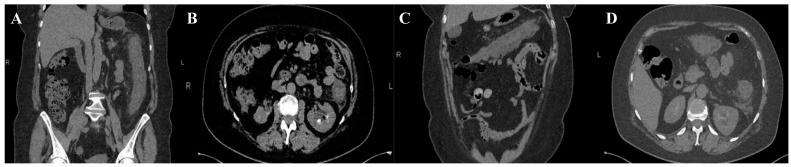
Second abdominal CT. Extensive and continuous lesions, up to hepatic flexure and down to sigmoid with pericolic fat stranding. Coronal (A,C) and axial planes (B,D) respectively.
